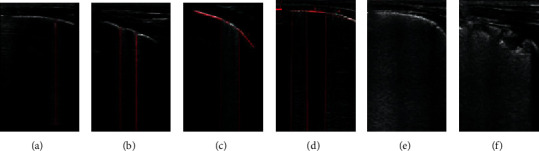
Display of pulmonary ultrasound data of typical cases.
